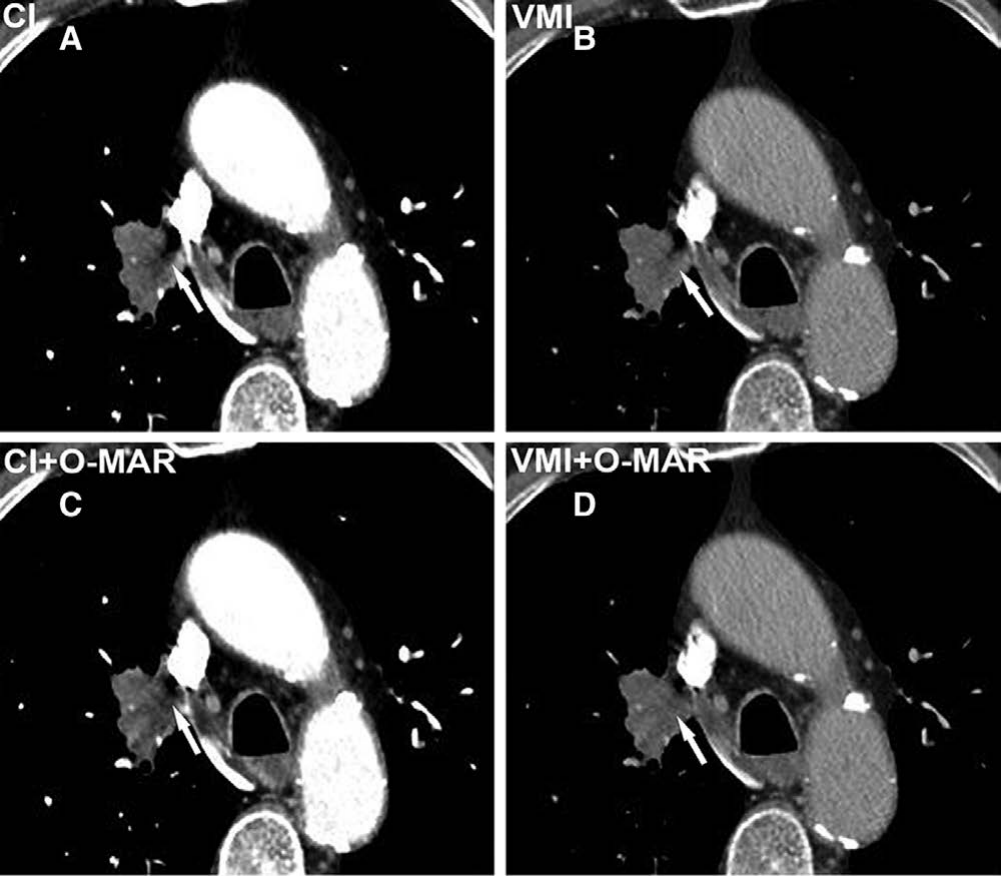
A 74-year-old man with lung cancer. Enhanced chest CT images show an irregular mass in the right lung near the mediastinum. On CI (A), it is hard to evaluate the intralesional density and the relationship with mediastinum due to the artifacts from CM in the superior vena cava (arrows). The VMI (B) and CI + O-MAR (C) reduce some artifacts and improve the image quality. The VMI + O-MAR image (D) shows a mass with homogenous density that has not invaded the mediastinum. Subjective assessments from both readers generated the best scores for the VMI + O-MAR image. CI = conventional images, CM = contrast media, O-MAR = orthopedic metal-artifact-reduction, VMI = virtual monoenergetic images.
